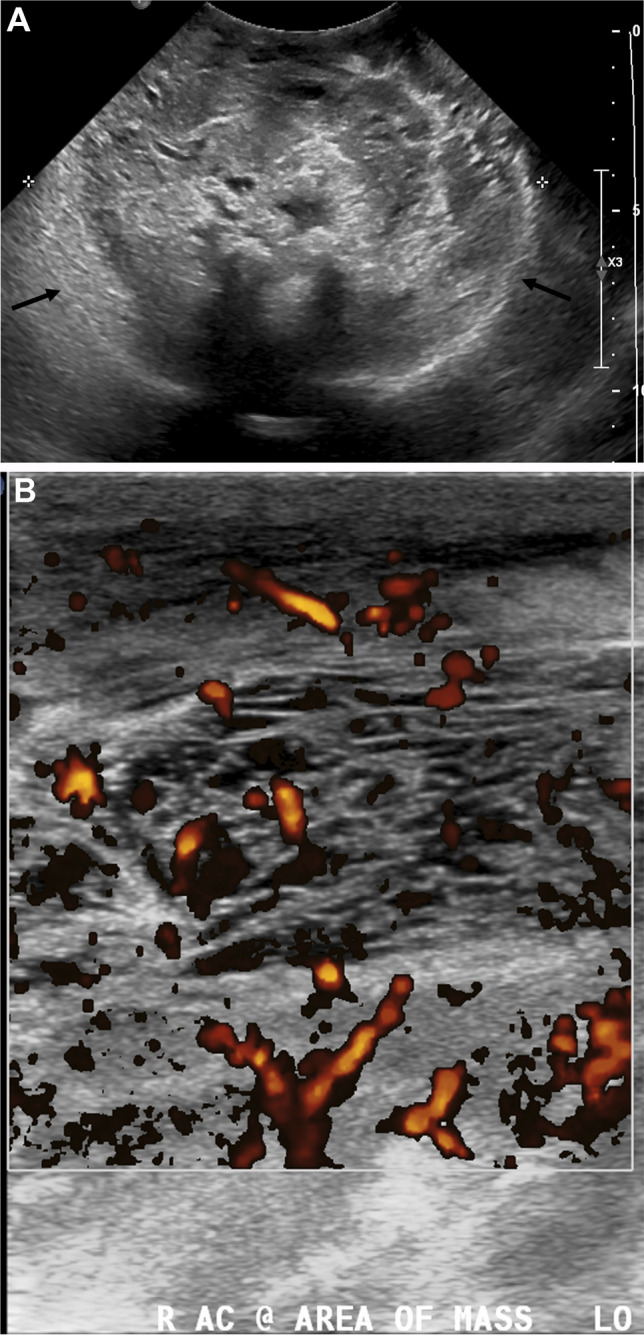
Gray scale (A) and Doppler (B) ultrasound images of the antecubital fossa demonstrate a large, heterogeneous, vascular mass (arrows in A)
